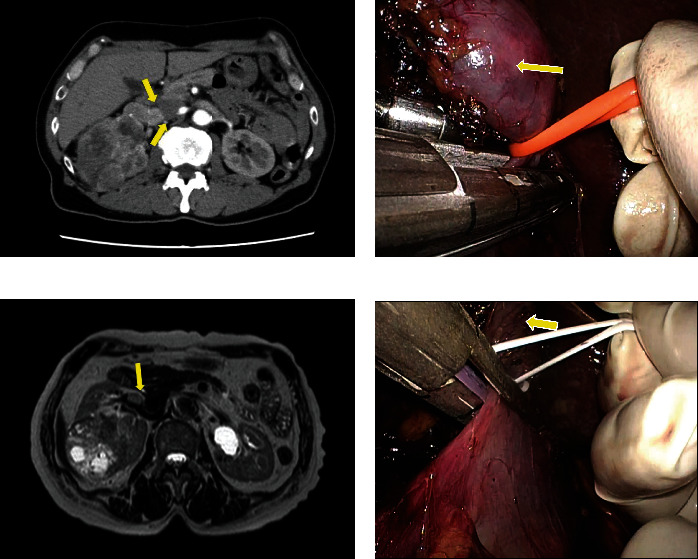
Imaging and intraoperative findings of the cases of right renal cell carcinoma (RCC) with thrombus ((a, b) case 1 and (c, d) case 2). (a) Computed tomography demonstrated a renal tumor thrombus extending 0.5 cm into the inferior vena cava. (b) The tumor was pulled up within the renal vein using the vessel loop, and the renal vein was divided using the Endo GIA stapler. (c) Magnetic resonance imaging demonstrated a renal tumor thrombus within the renal vein. (d) The tumor was pulled up using the vessel loop, and the renal vein was divided using the Endo GIA stapler. Arrowheads indicate the tip of the tumor thrombus.
